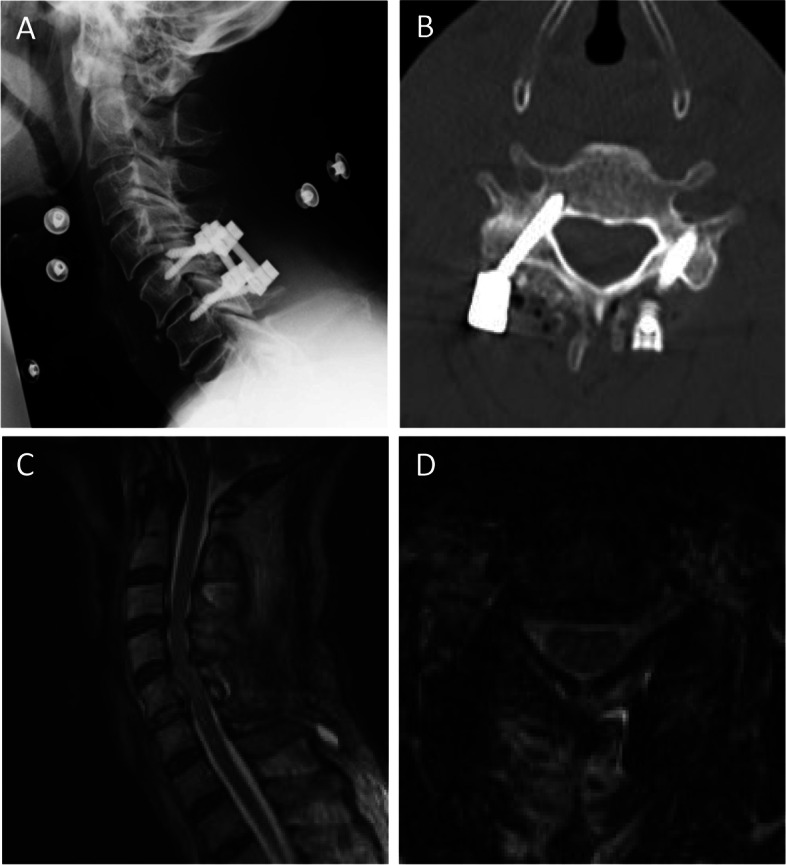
Postoperative X-ray (A), CT (B), MRI (C and D) shows successful reduction of dislocation, instrumentation, and decompression of the spinal cord
